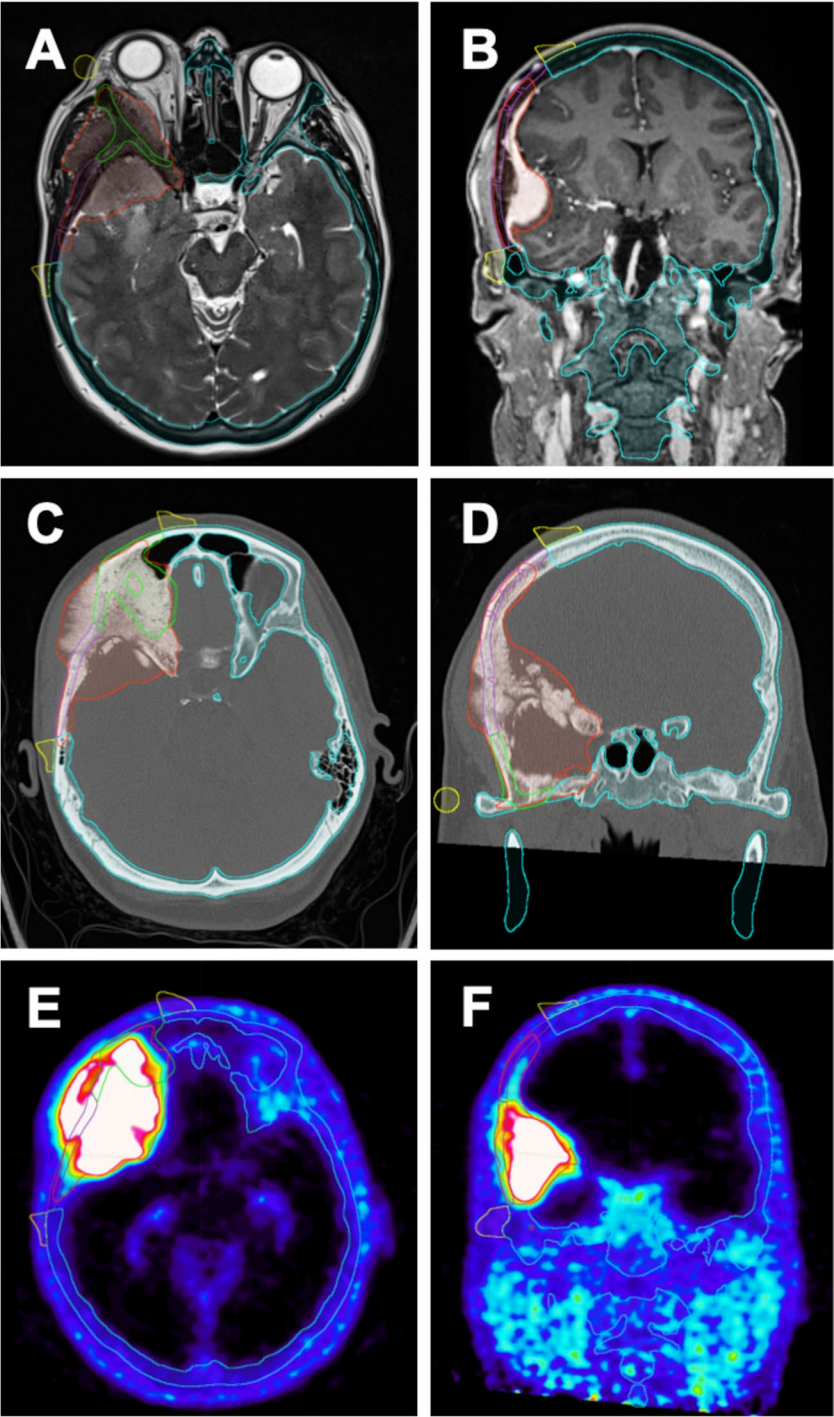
Preoperative virtual planning in Brainlab Elements software, using MRI T2 and contrast enhanced T1 sequences (A&B), cranial CT scans (C&D) and 68Ga-DOTATOC PET/CT scans (E&F). Tumor margins are defined by the treating surgeons (red lines), bony resection margins are defined (blue lines) and externally planned cutting guides (yellow lines) and implant margins (green lines) are later merged as STL files into the Brainlab planning (A-F)
